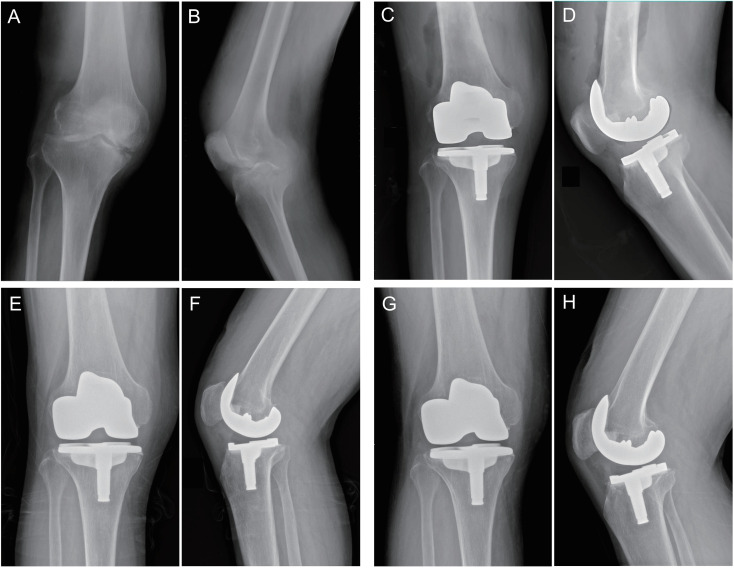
Serial radiographs of the right knee. (A, B) Preoperative anteroposterior (AP) and lateral views. (C, D) Postoperative radiographs at 3 months. (E, F) Postoperative radiographs at 12 months. (G, H) Postoperative radiographs at 24 months, demonstrating satisfactory implant positioning.
